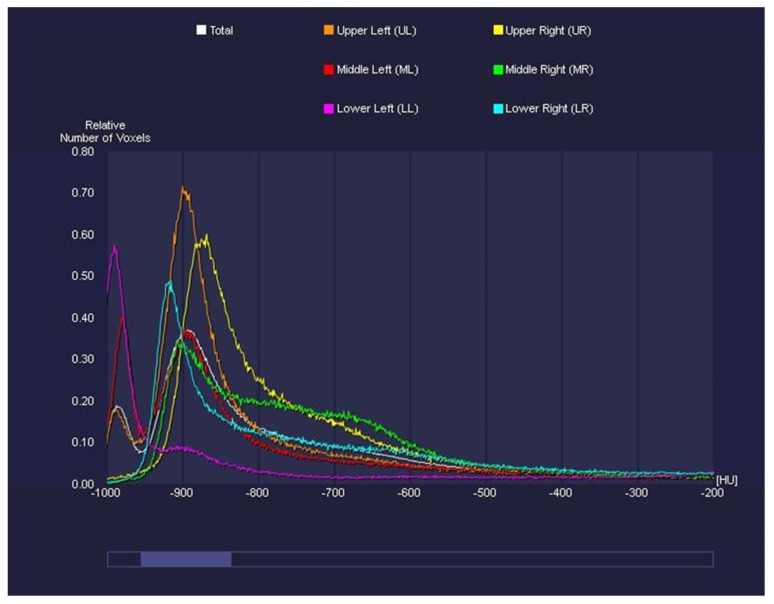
CT Pulmo 3D: graphic of HU thresholds. This graphic represents the HU thresholds and their relative frequencies.
